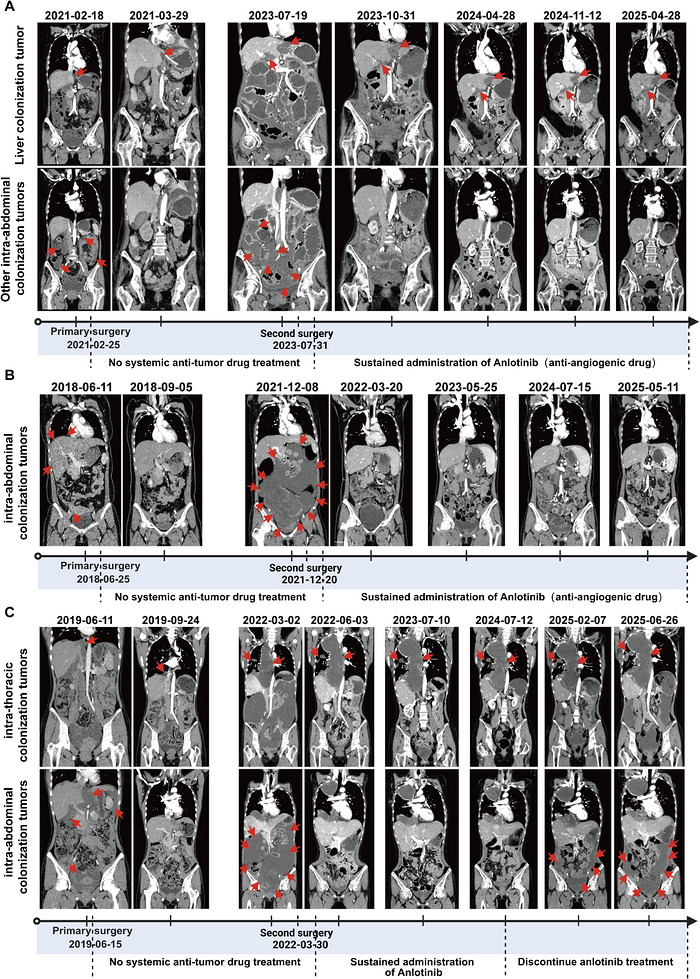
Clinical course of three patients with recurrent PMP treated with anti‐angiogenic therapy. (A‐C) Treatment timelines and representative CT images from three patients with recurrent PMP who received anlotinib after secondary CRS. In patients 1 and 2 (A and B), sustained anlotinib administration after secondary surgery was associated with prolonged radiological disease control until the last follow‐up. In patient 3 (C), the disease remained radiologically stable during anlotinib treatment but progressed after treatment discontinuation. Red arrows indicate low‐density metastatic lesions of PMP.
